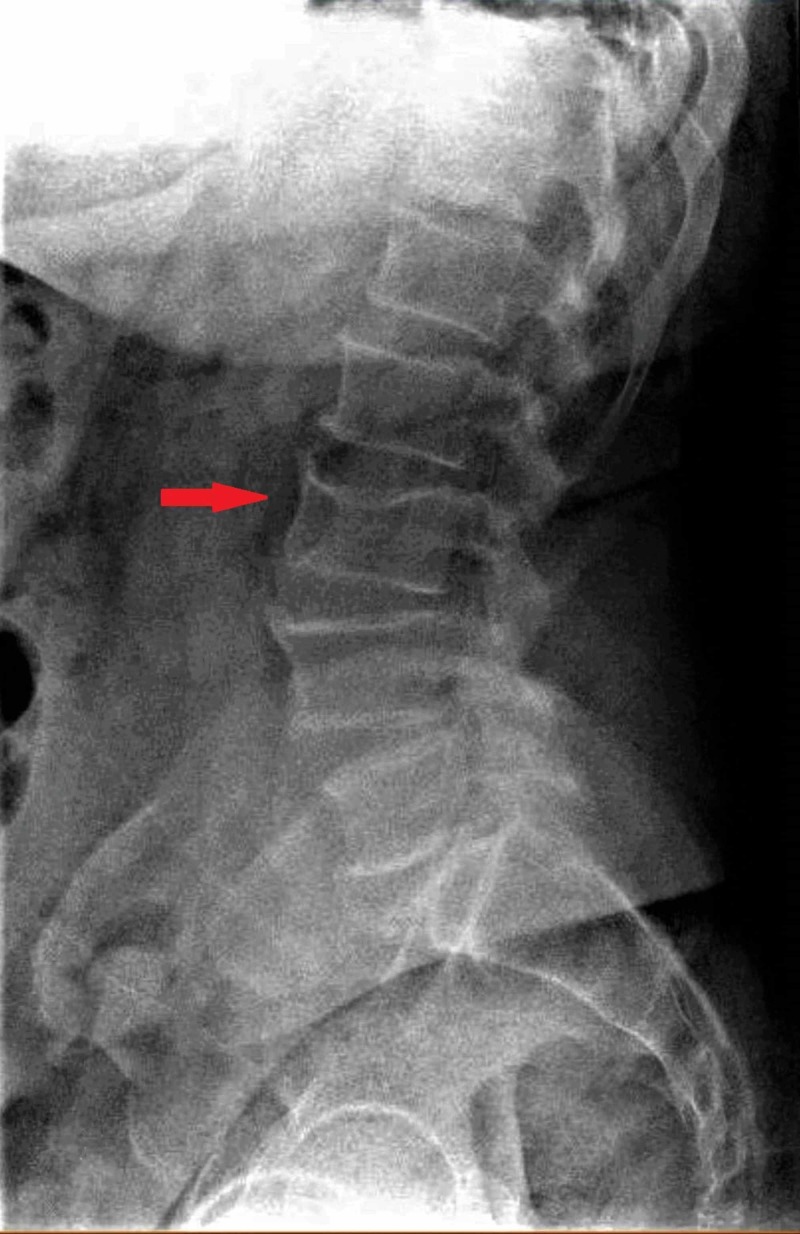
Lateral view of the lumbar spine X-ray showing L3 vertebral deformity
